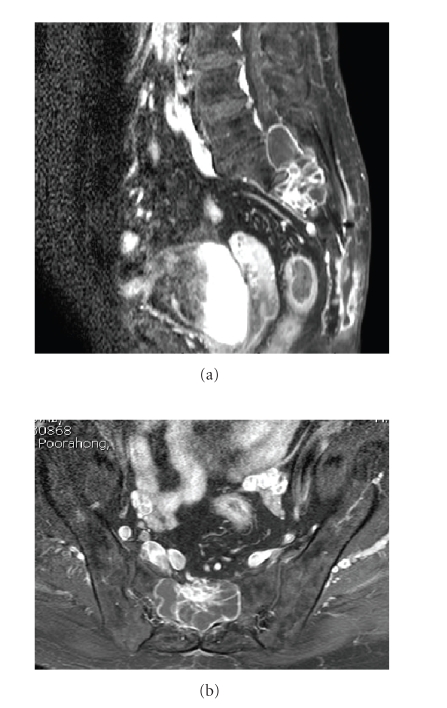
Sagittal (Figure 3(a)) and axial (Figure 3(b)) T1-weighted/GD/FS MR
